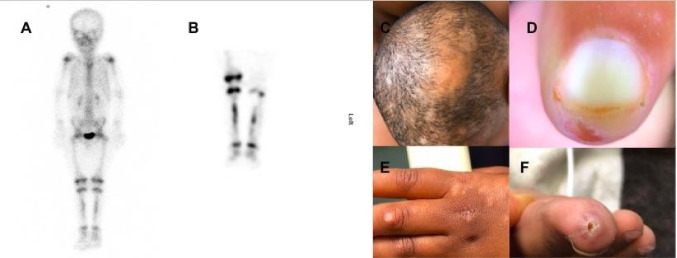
Baseline clinical and imaging findings in patient 2. A Whole-body scintigraphy showing increased radiotracer uptake in the upper limb musculature, chest wall, abdominal wall, elbow and wrist joints, and thighs. B SPECT imaging further confirming focal muscle inflammation with increased uptake. C Patchy alopecia. D Vasculitic lesion on the distal phalanx of the finger. E Gottron’s papule on the interphalangeal joint along with hypochromic lesions. F Vasculitic ulcerative lesion
